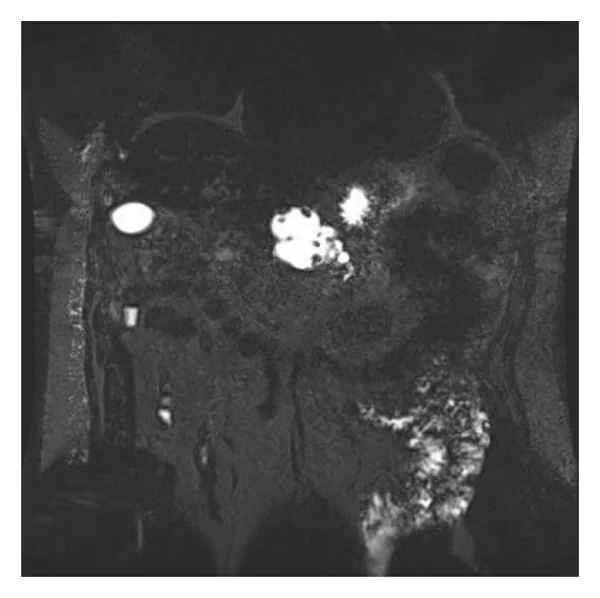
The patient’s MRI in the coronal plane with a 5.0 × 3.9 × 3.8 cm cystic mass with multiple enhancing mural nodules.
